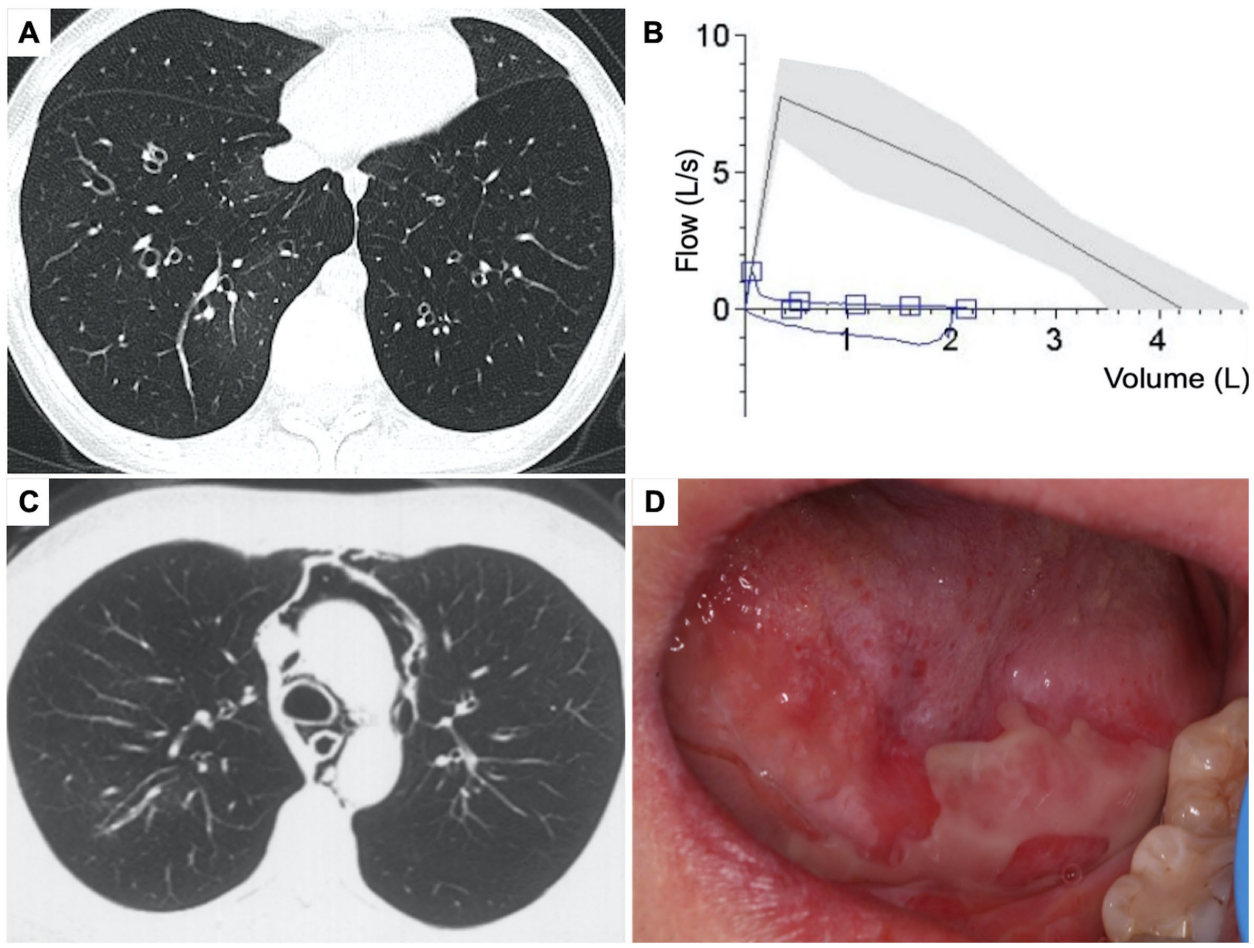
Clinical presentation of the patient. (A) Chest high resolution CT, axial view showed diffuse bronchiectasis with patchy ground-glass opacity and pulmonary hyperinflation. (B) Pulmonary function test showed severe obstructive dysfunction. (C) Chest CT showed spontaneous pneumomediastinum. (D) Physical sign of oral mucosal erosions on the tongue.
